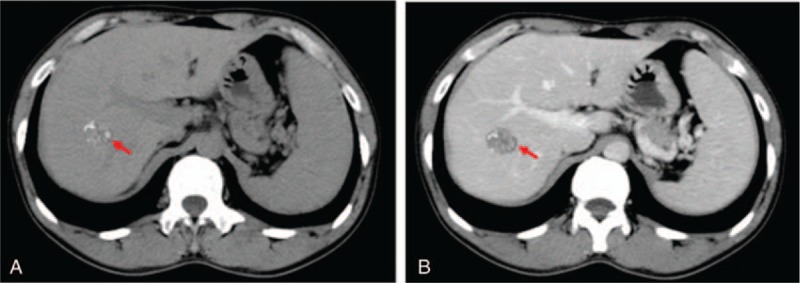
The preoperative upper abdominal CT for the HAE patients. A, Unenhanced CT. B, Enhanced CT. The red arrow indicates the HAE lesion. HAE = hepatic alveolar echinococcosis, CT = computed tomography.
